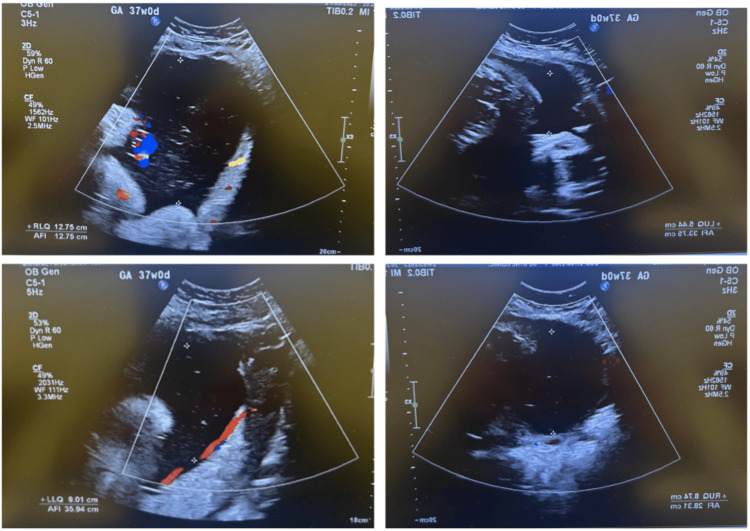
Ultrasound image showing polyhydramnios at 37 weeks Follow-up ultrasound at 37 weeks showing progression of polyhydramnios (AFI: 35.9 cm) AFI: amniotic fluid index
